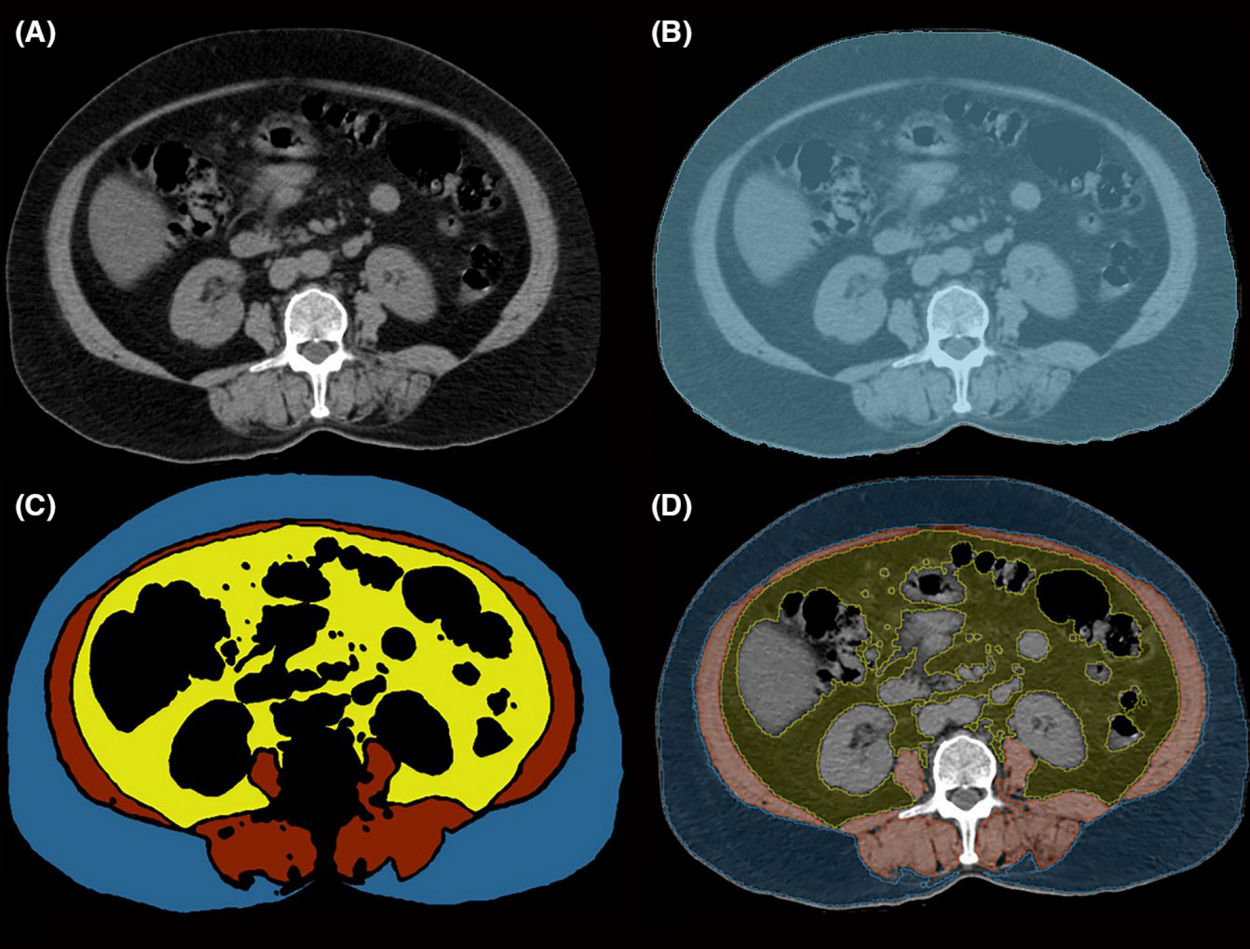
Axial unenhanced CT scan at L3 vertebrae mid‐corpus level. (A) raw image; (B) all tissues segmented (single slice); (C) Mask images of segmentation, blue represents subcutaneous fatty tissue, red muscle tissue and yellow mesenteric fatty tissue; (D) Segments on raw CT image. CT, computed tomography.
